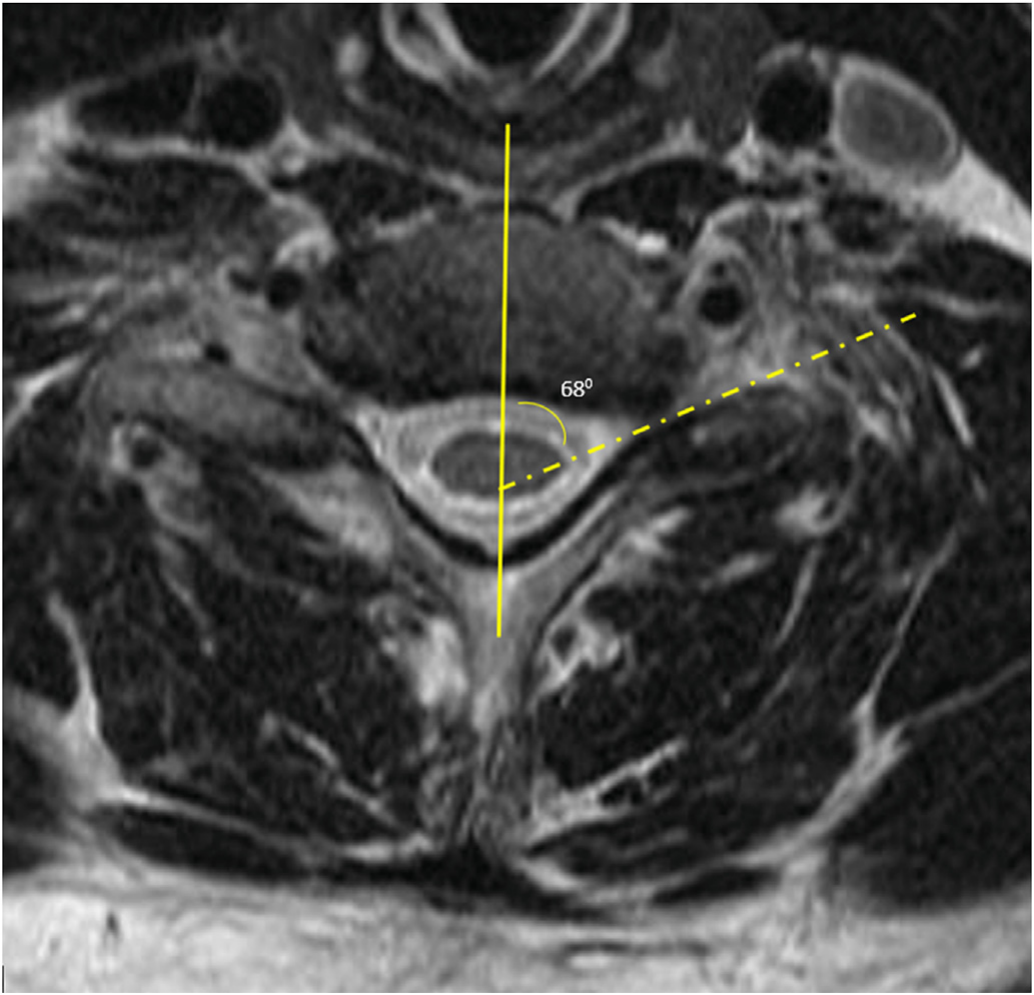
T2 Axial MRI measurement of the left C7 superior articular process ventral surface angle in preparation for a Left C6/7 TFESI. Initially a line is drawn in the sagittal midline bisecting the disc and spinous process (solid line). A second line is drawn along the ventral surface of the SAP (dashed line) to intersect with the sagittal line. The angle is measured (in this case, 68°) using the MRI angle measurement software.
